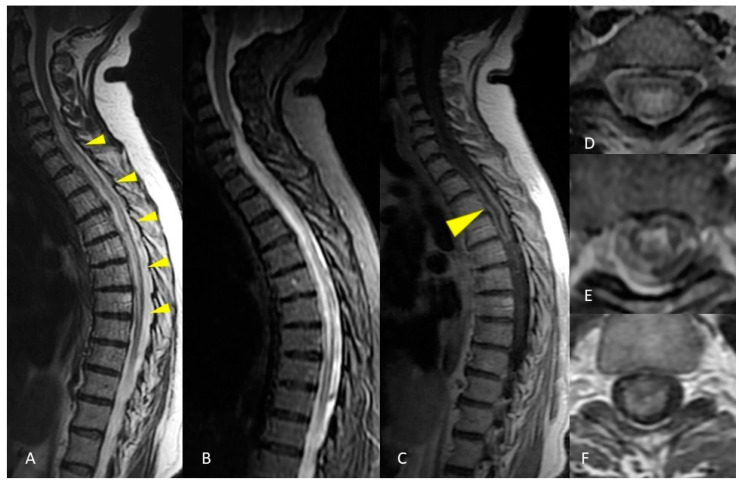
MRI of the spinal cord. Sagittal T2-weighted and STIR images show a long and continuous segment of abnormal hyperintensity affecting the cervico-thoracic spinal cord (A,B). Axial T2-weighted image shows diffuse hyperintensity involving all the cross-sectional area of the spinal cord at a more cranial level (D) and a predominant gray matter involvement at a more caudal level (E). Spinal cord swelling and enhancement were present at the segmental thoracic level, evident in both sagittal (C) and axial (F) images.
